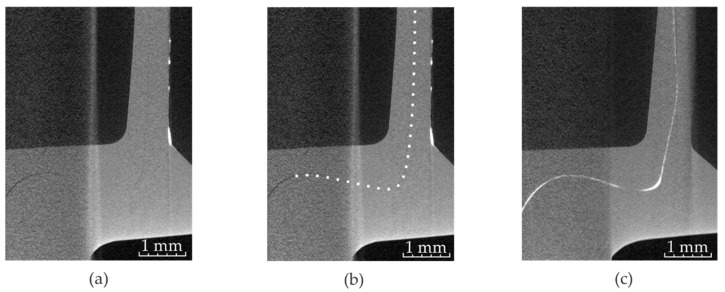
Detail of a computed tomography (CT) image of a clinch point with poorly visible sheet–sheet interface (a) and the same CT image with the assumed interface marked with a white dotted line (b). The same detail with copper varnish (applied on both sheets) in the sheet–sheet interface (c).
